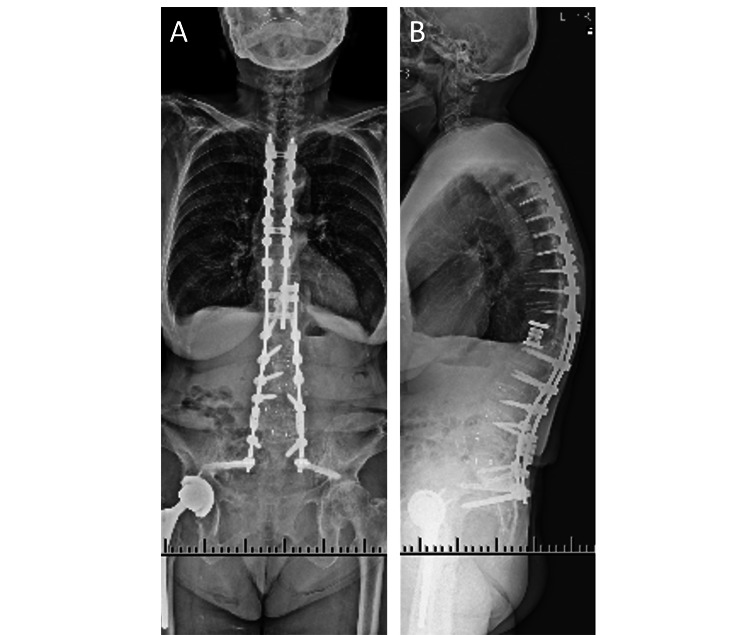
Final follow-up images. (A,B) After 2-year revision surgery, radiographs showed bone fusion at L5, and alignment was maintained.
